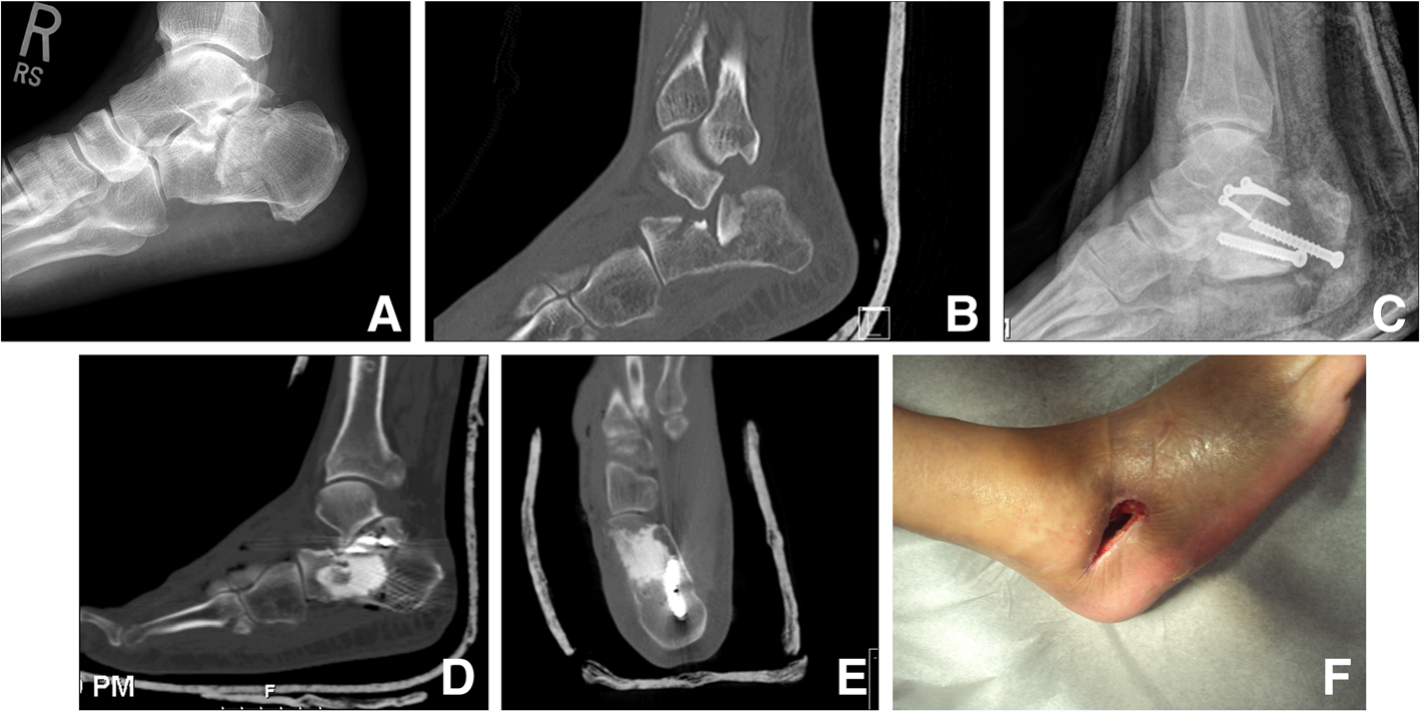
61 years old male, who had right calcaneus fracture with depression of the intra articular joint. A, B the injury radiograph and Sagittal CT showing the injury with severe comminution and depression. Two days after the injury, patient had open reduction using sinus tarsi approach with internal fixation using two cannulated 6.5 mm screws from the inferior aspect of the calcaneus tuberosity and three cortical screws supporting the depressed subtalar fragment. Synthetic bone grafting by Calcium Phosphate was used to fill the gap after elevating the depression. C, D and E: post operative radiograph, sagittal and coronal CT showing the reduction, fixation and grafting of the fracture. At the three weeks follow up, the patient presented with wound dehiscence and infection. Surgical debridement was performed and negative pressure wound therapy was applied until the wound healed with secondary intention to allow for regeneration of the deep defect. F: shows wound dehiscence under treatment by negative pressure wound therapy.
